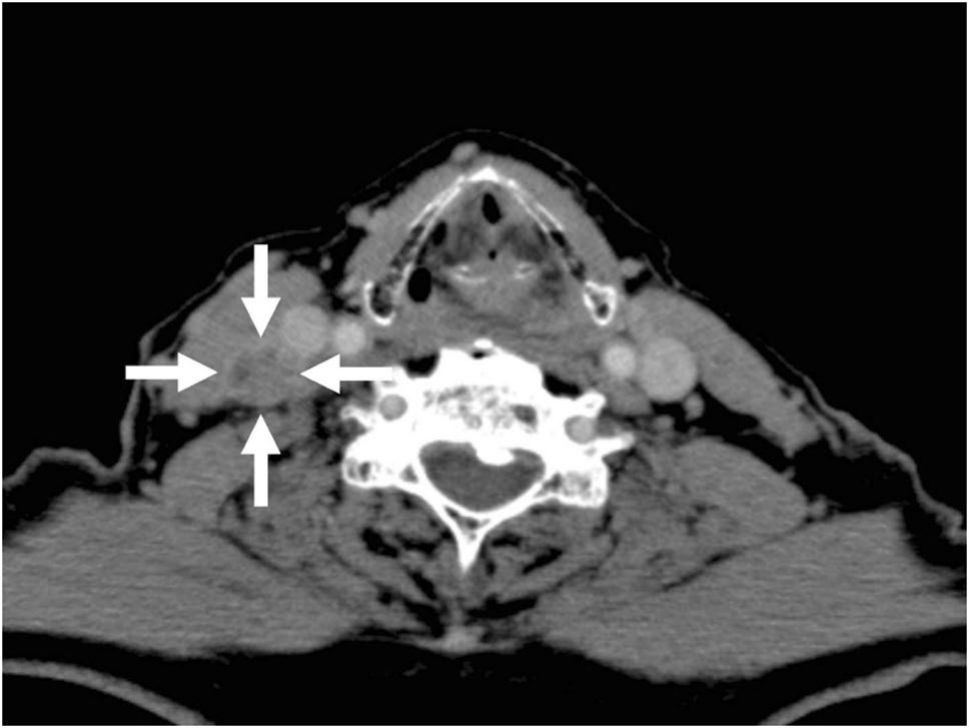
Contrast-enhanced CT 6 months after tumor resection showed a new swelling (arrows) of the right upper cervical lymph node, suggesting metastasis
